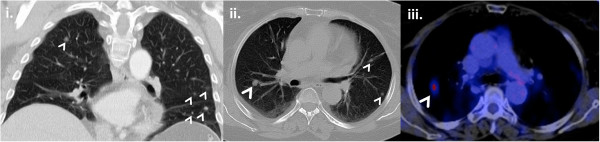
Imaging of the bilateral pulmonary nodules at initial evaluation. i. and ii. Multiple bilateral pulmonary nodules (white arrows) in CT imaging iii. High uptake of ^18^ F-FDG in one pulmonary nodule (white arrow) (SUV of 3.7), highly suspicious for malignancy.
